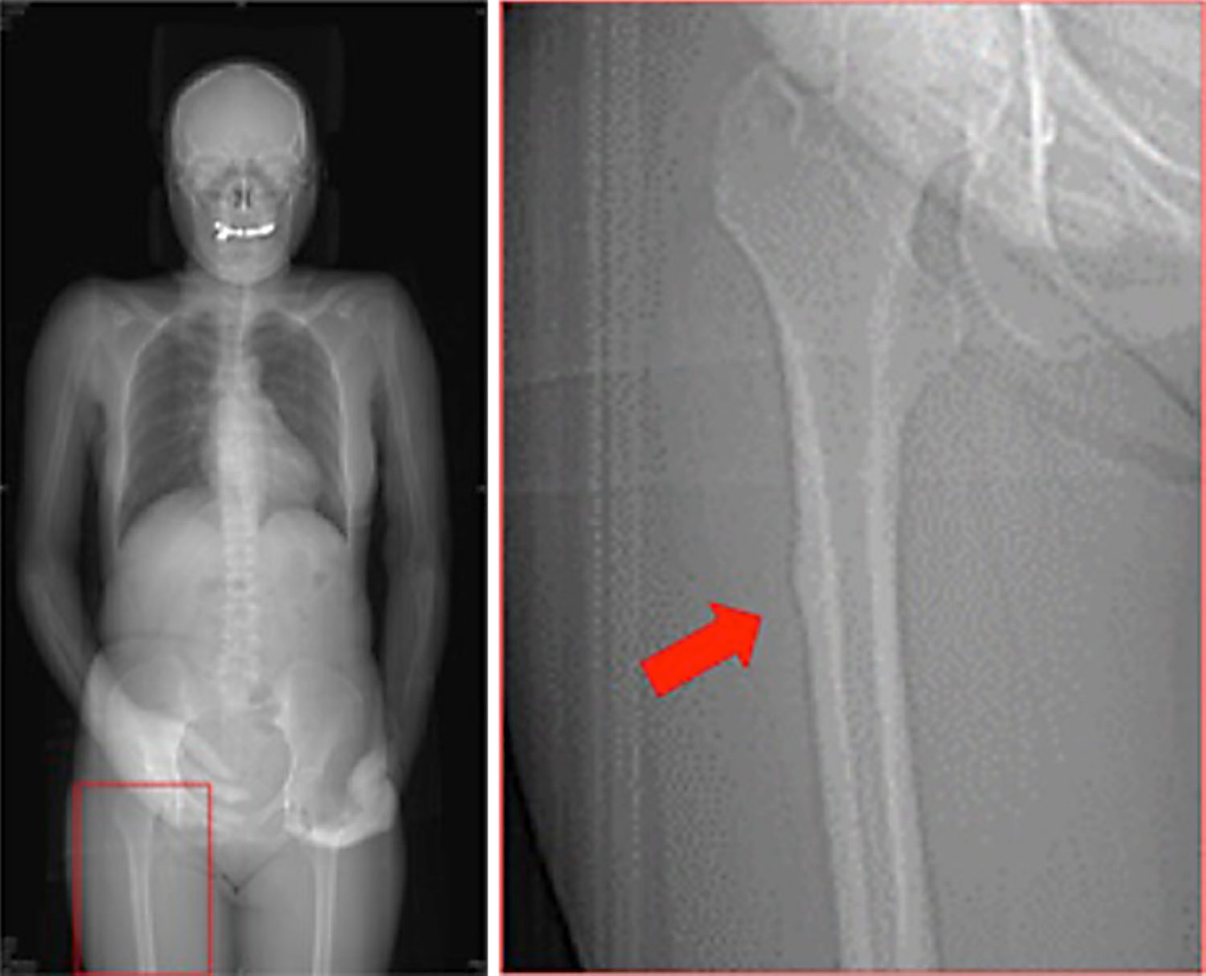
Beaking of lateral cortex in scout view of CT. The presence of localized periosteal or endosteal thickening of the lateral cortex at the subtrochanteric region (beaking) in CT scout view
